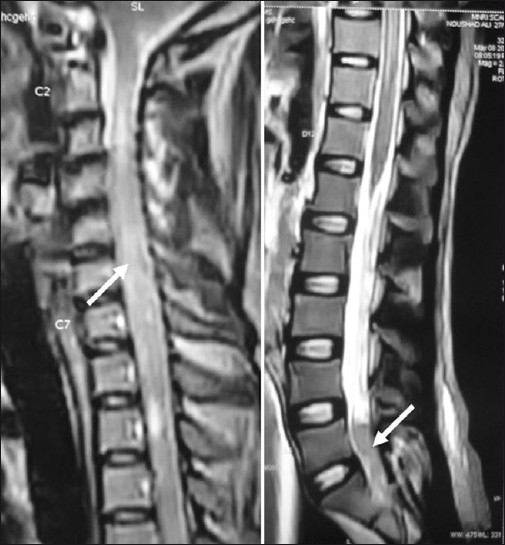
Saggital MRI of the cervical and sacral region showing drop metastasis (shown by arrows)
